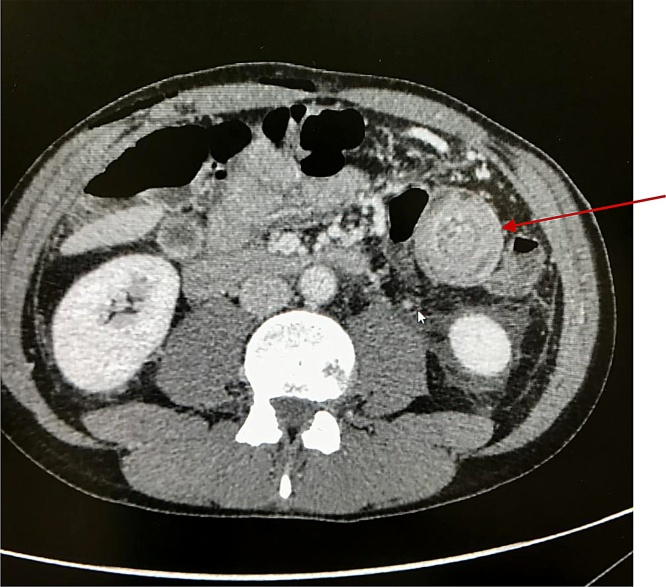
CT scan image: The arrow indicates invagination of intestinal loop in the left hypochondrium with endolume corpuscular fluid component.
